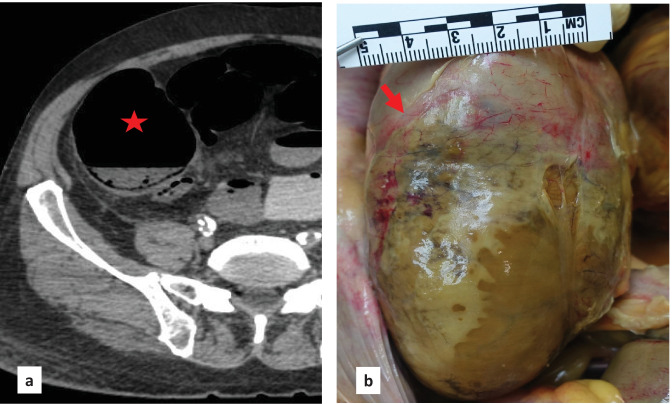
a Post-mortem CT-scan showing dilation of the cecum (red star). b Autopsy in situ view of the anterior part of the cecum presenting diffuse green-brownish discoloration with a red–purple well delineated edge (red arrow)
